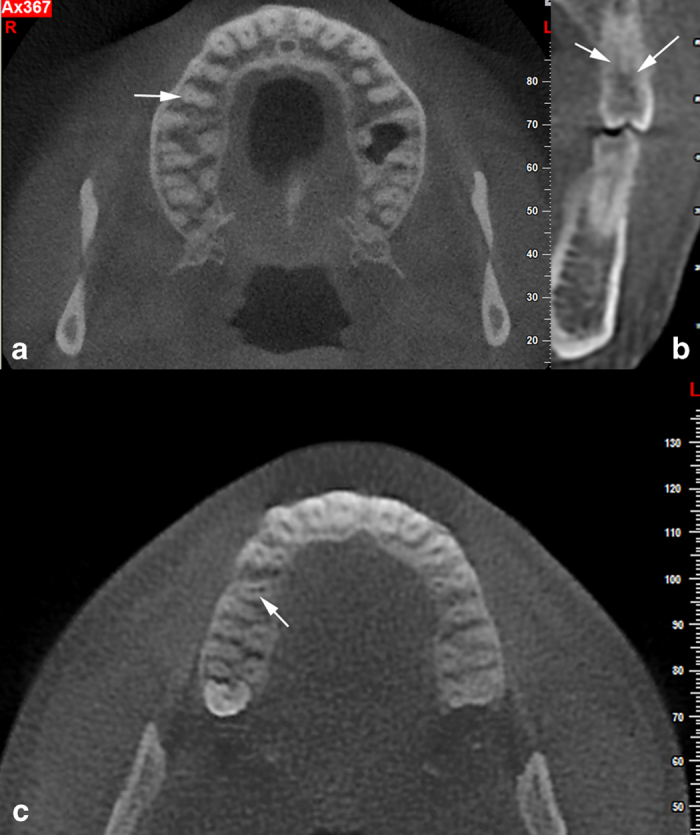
(a, b) Axial and cross-section CBCT images showing two rooted two canals maxillary second premolar. (c) Axial CBCT image showing type II maxillary second premolar (arrows).
